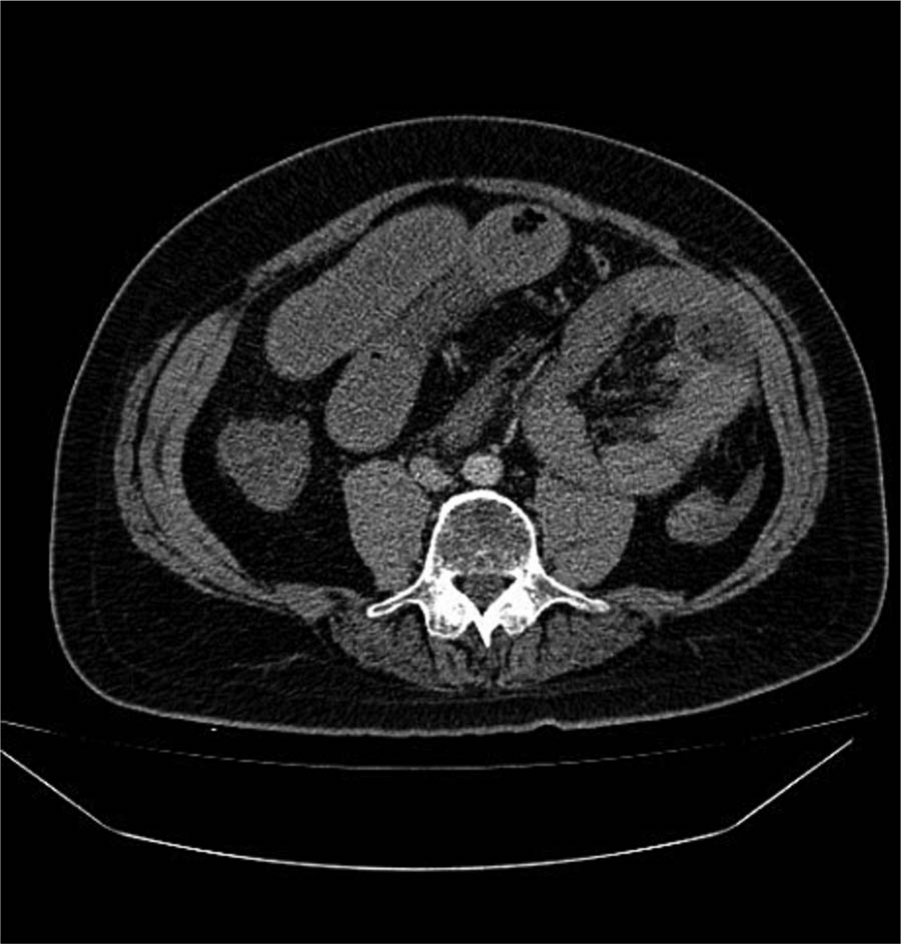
Contrast-enhanced axial CT abdomen: cluster of jejunal loops in the left upper quadrant forming a sac beneath the SMV, with proximal small-bowel dilatation consistent with closed-loop obstruction.
